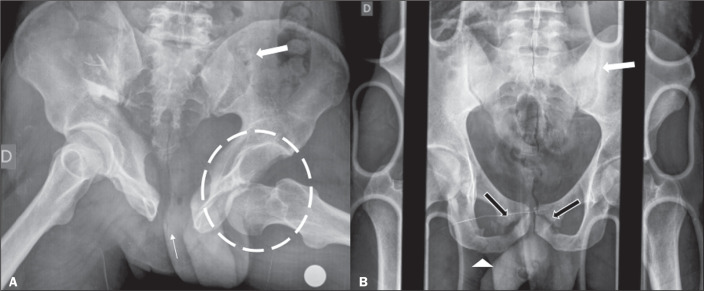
A: Anteroposterior X-ray of the pelvis of a 39-year-old man who had
